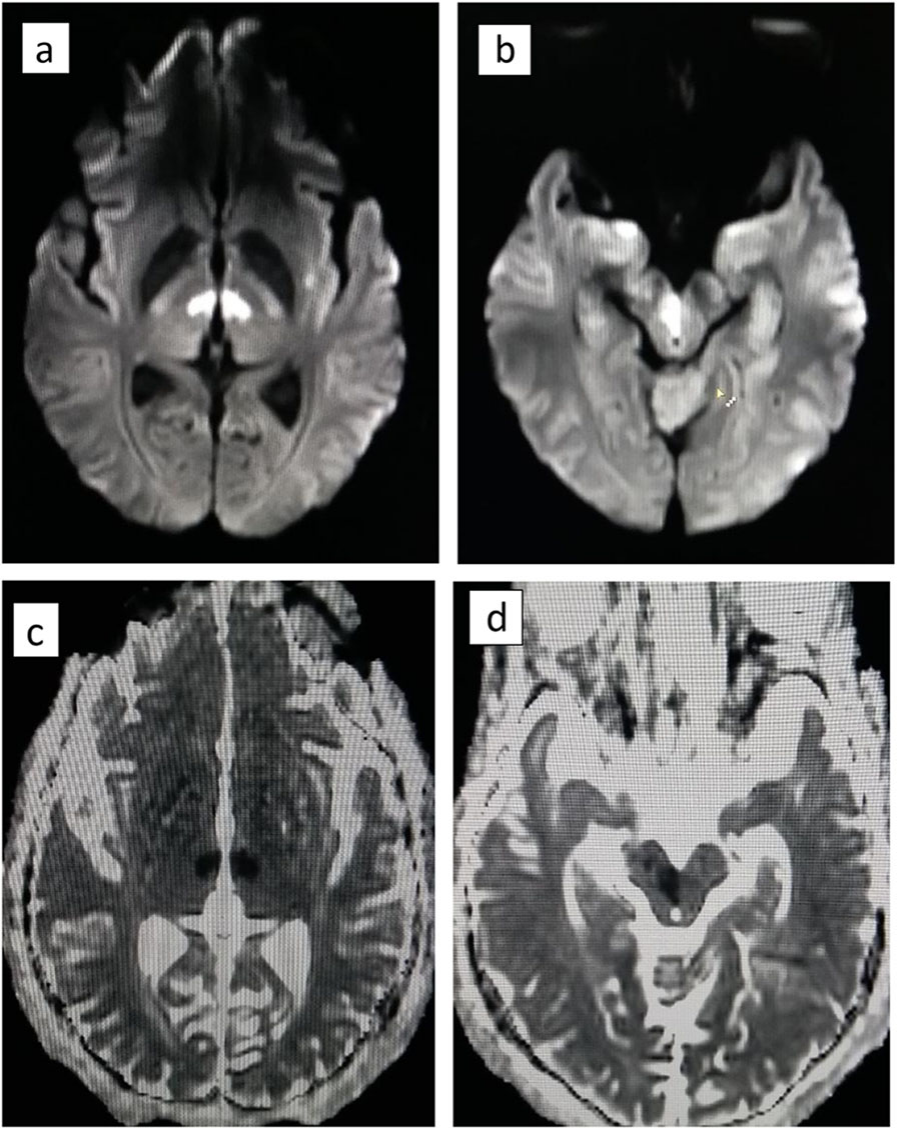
MRI images showing AOP territory infarction. a DWI image showing paramedian thalamic infarctions. b DWI image showing right rostral midbrain infarction (Note the assymetry in rostral mid brain involvement). c ADC image showing paramedian thalamic infarctions. d ADC image showing assymetrical right rostral midbrain infarction
